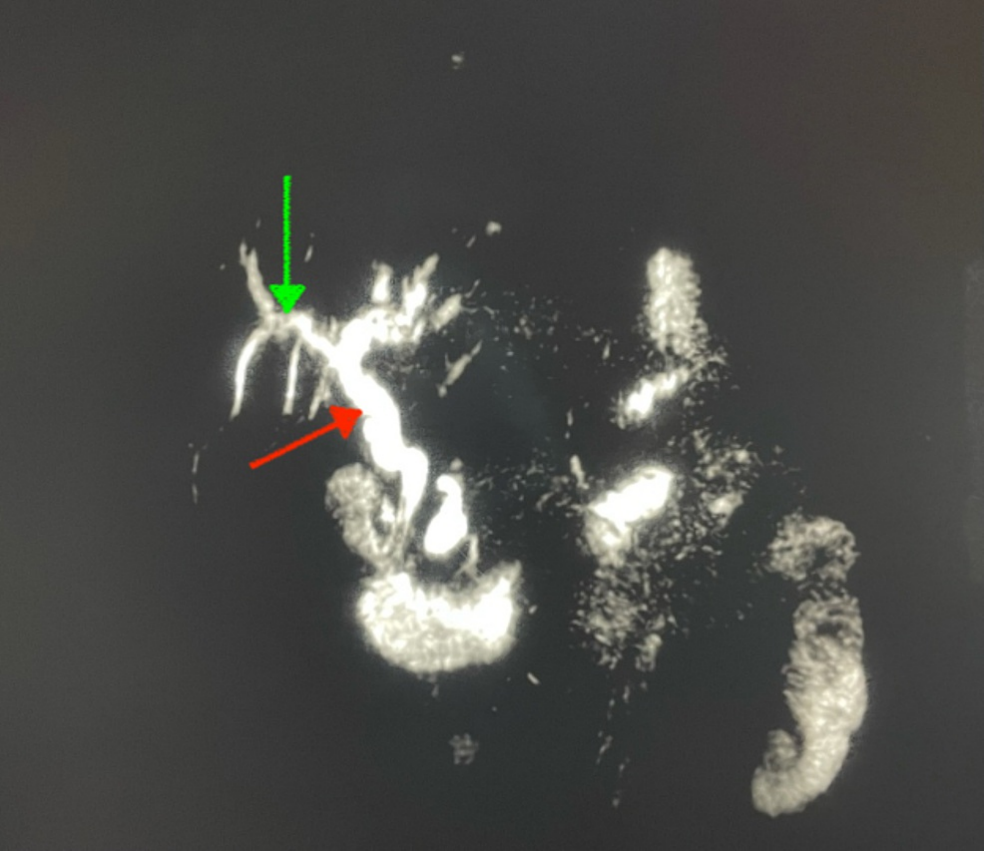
A 70-years-old patient with cholangitis: 3D MRI sequences and showing segmental dilation (red arrow) of the intra and extrahepatic bile ducts (green arrow). MRI: magnetic resonance imaging.
